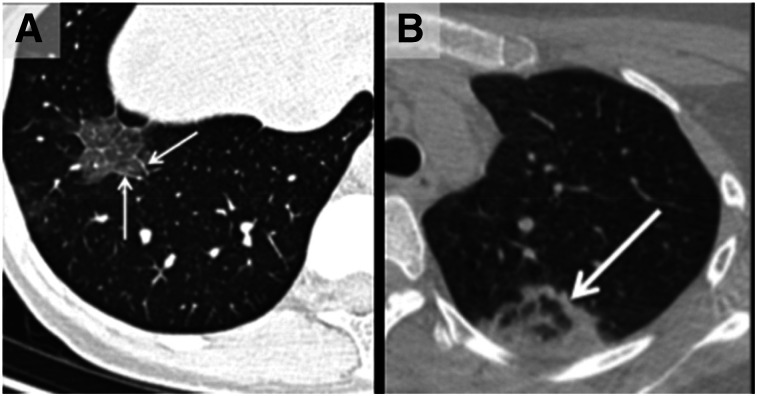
(A) Unenhanced axial CT image from 43-y-old woman shows crazy‐paving pattern as manifested by right-lower-lobe ground‐glass opacification with interlobular septal thickening (arrows) and intralobular lines. (B) Axial CT image from 22‐year-old woman shows area of faint ground‐glass opacification in left upper lobe, with ring of denser consolidation (arrow, reverse halo sign). (Reprinted with permission of (32).)
